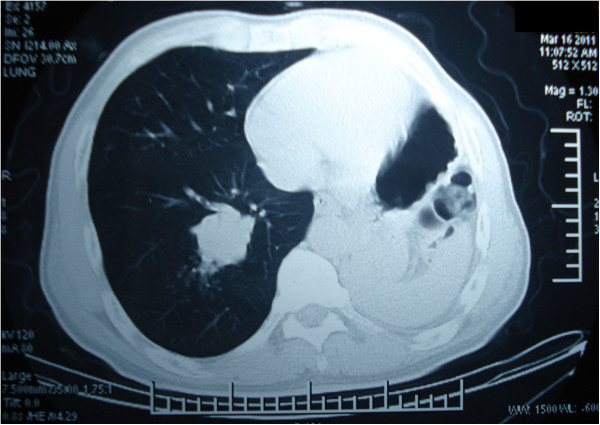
Preoperative chest CT showing a 4 cm mass in the right lower lobe. There is favorable hyperinflation of the right lung and normal postpneumonectomy status.
